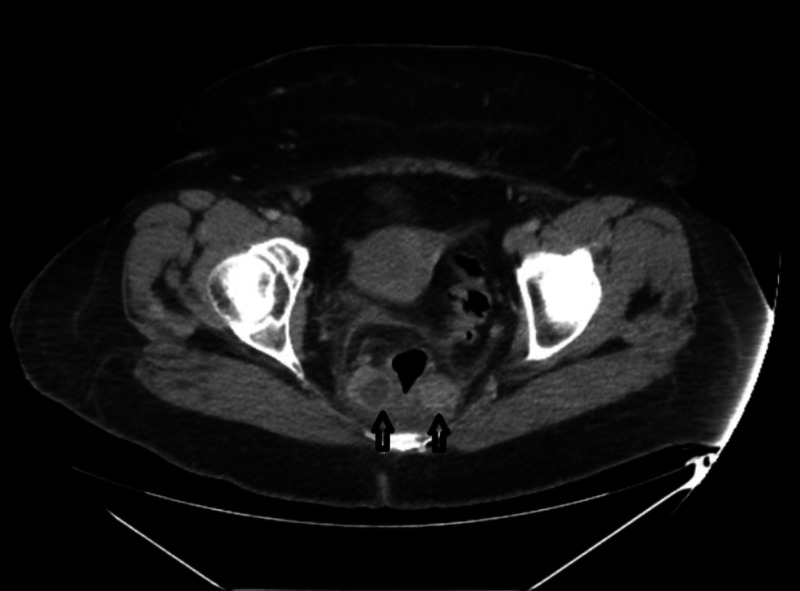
CT scan of the pelvis. Perirectal: 17-mm perirectal abscess to the right of the rectal vault and a soft tissue density in presacral space (arrows).
